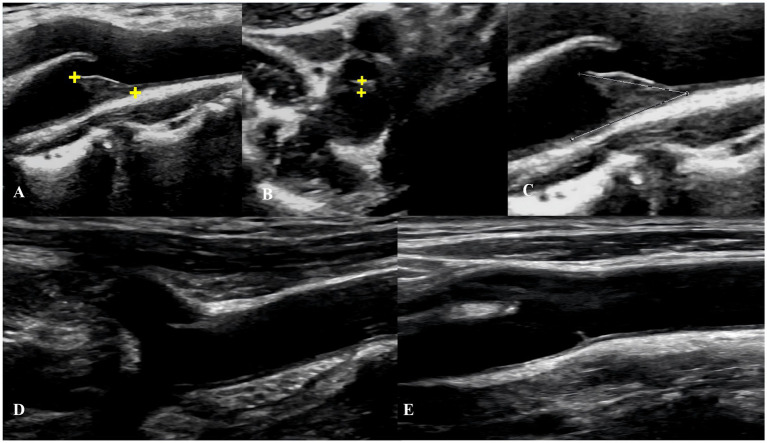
Measurements and morphological types of carotid web on ultrasound images. (A) Measure the length of the carotid web on the longitudinal section, measuring the distance between the tip of the carotid web and the origin of the base of the carotid web. (B) Measure the thickness of the carotid web at a point where the thickness is relatively uniform on the cross-section. (C) Measurement of carotid web angle using Adobe Photoshop CS3. (D) Representative longitudinal B-mode image of a curved CW. (E) Representative longitudinal B-mode image of a straight CW.
